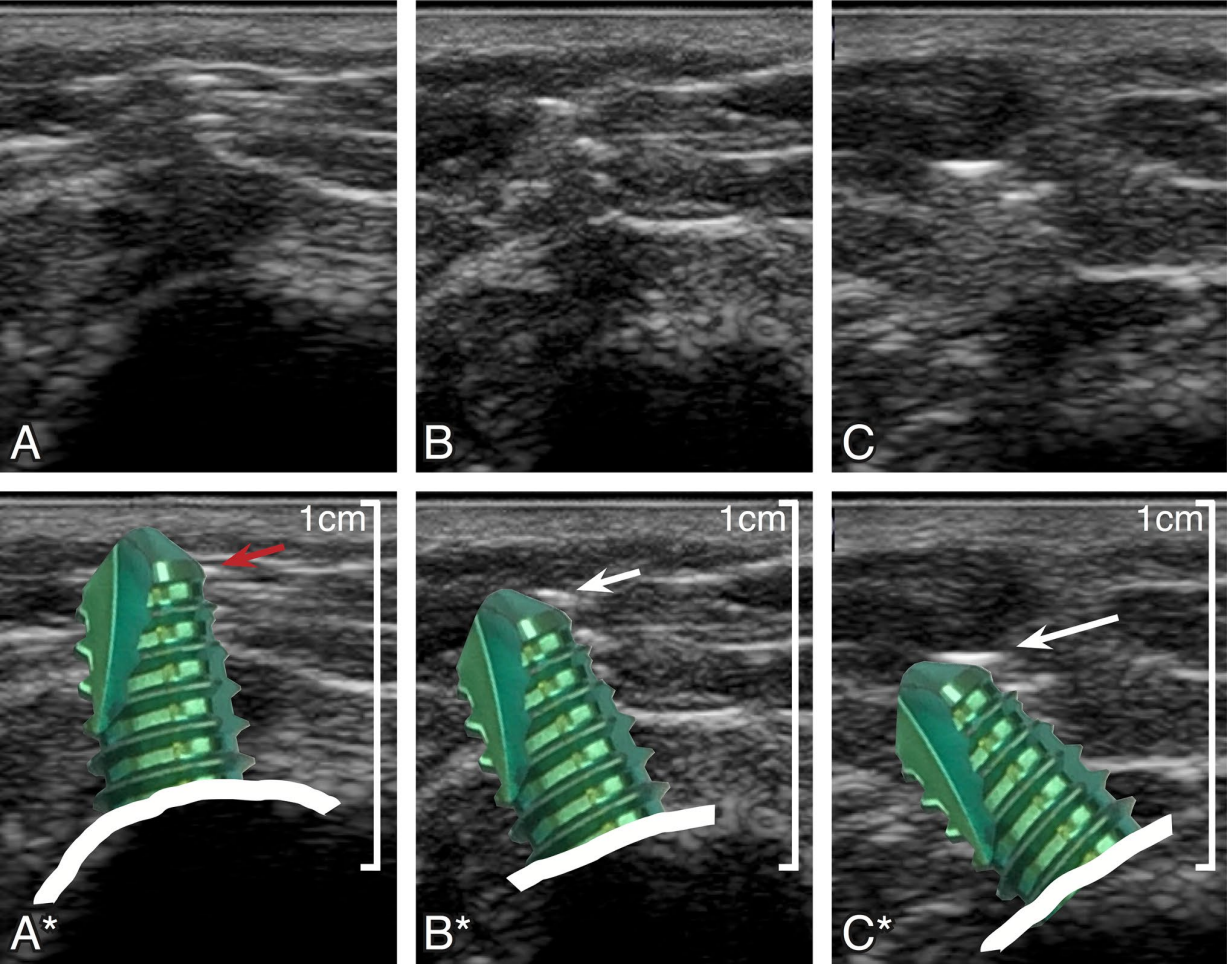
Different reflection patterns of the screw tip dependent on the angle of the ultrasound beam. Lateral tilting of the ultrasound beam (B/B* and C/C*) helps to better visualize the most superficial end of the screw tip. To obtain an oblique scan of the screw may help to improve visibility. The better the cone surface of the screw tip is rectangular orientated to the ultrasound beam, the brighter the reflection (white arrow) and the better the visibility of the real tip of the screw will be. By scanning more or less in the direction of the screw (A and A*) there might be only little reflection on the rounded tip of the screw. It is more likely to get the first bright reflection on the first thread (red arrow) than the tip of the screw (color figure online)
